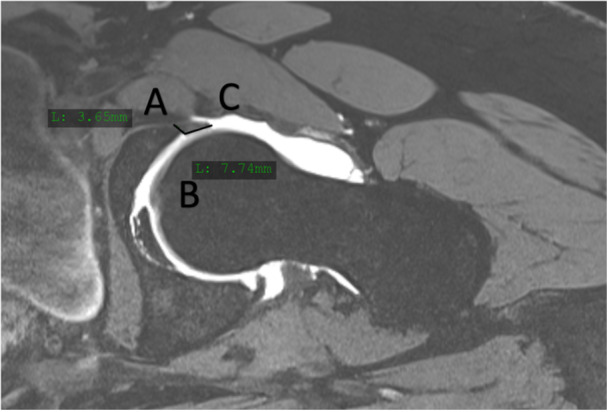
Examples of anterosuperior labral hypertrophy (height [AB] to length [AC] ratio ≤1:2) measured on axial MRI imaging.
